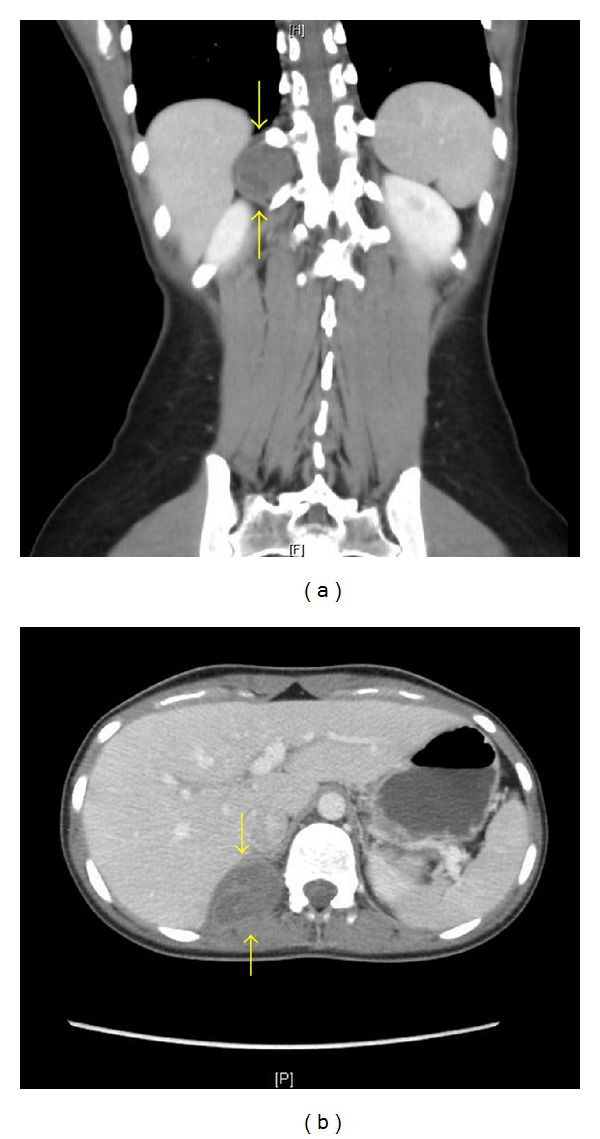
Coronal (a) and transverse (b) contrast-enhanced computed tomography (CT) scans showing a 4.2 × 3.2 cm, heterogeneous noncalcified mass involving most probably the right adrenal gland region (arrows).
